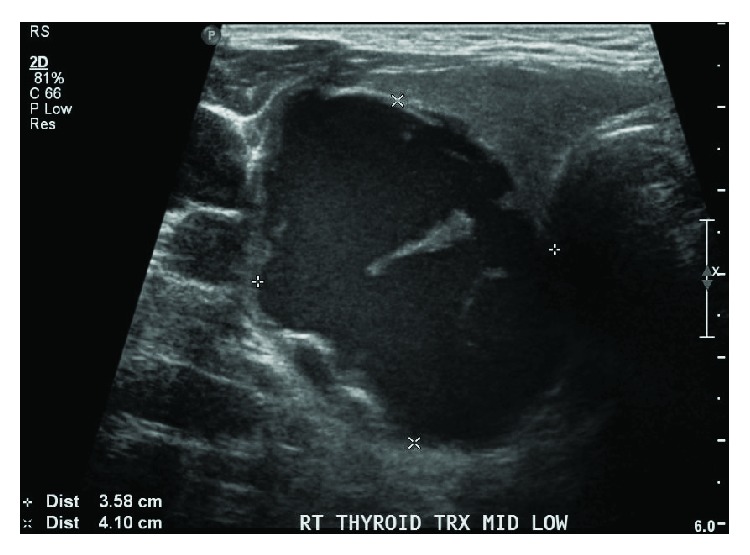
Ultrasound showing the parathyroid mass.
